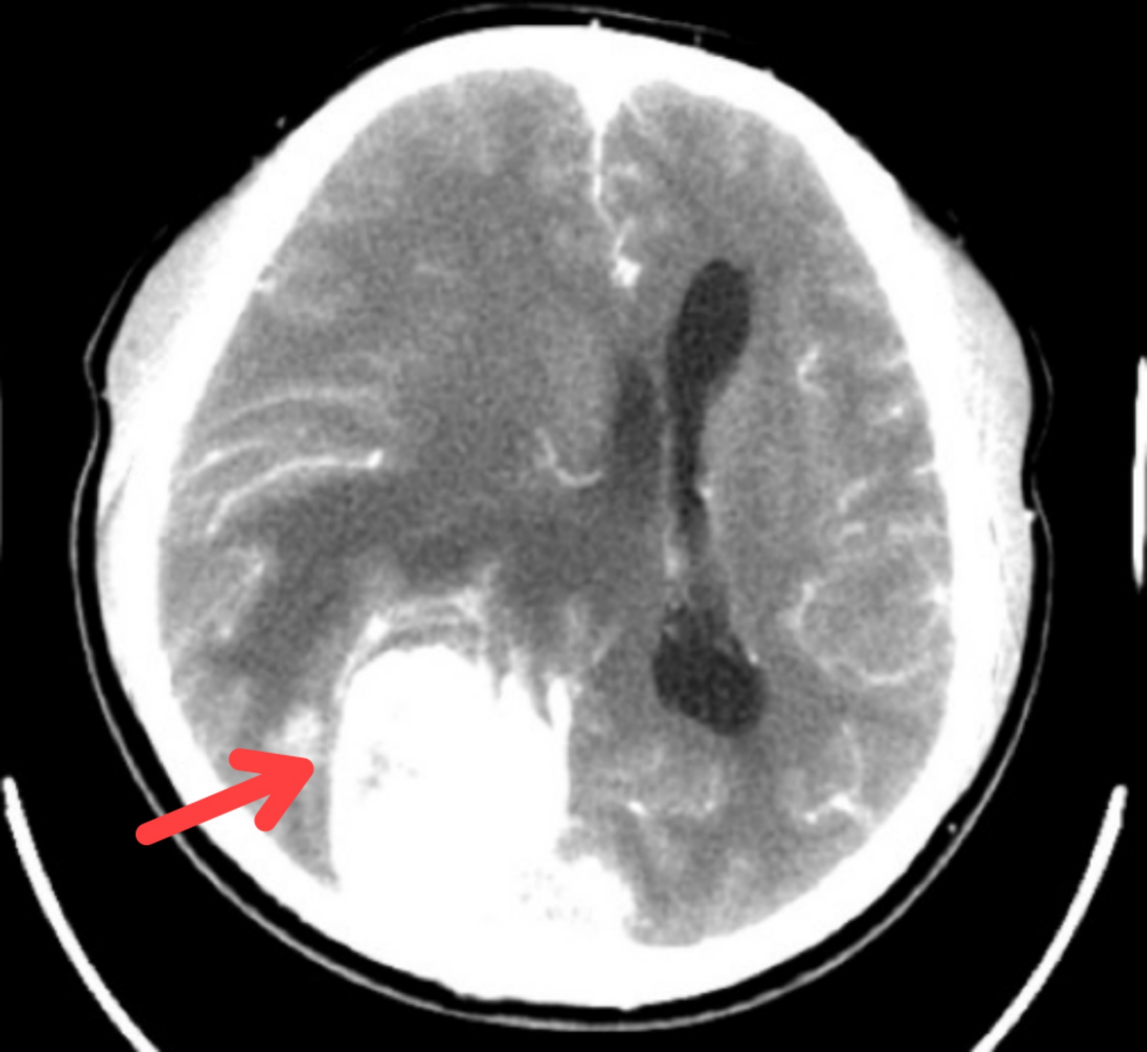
Computed tomography scan with contrast during first presentation showed a huge right occipital lobe tumour with mass effect.
